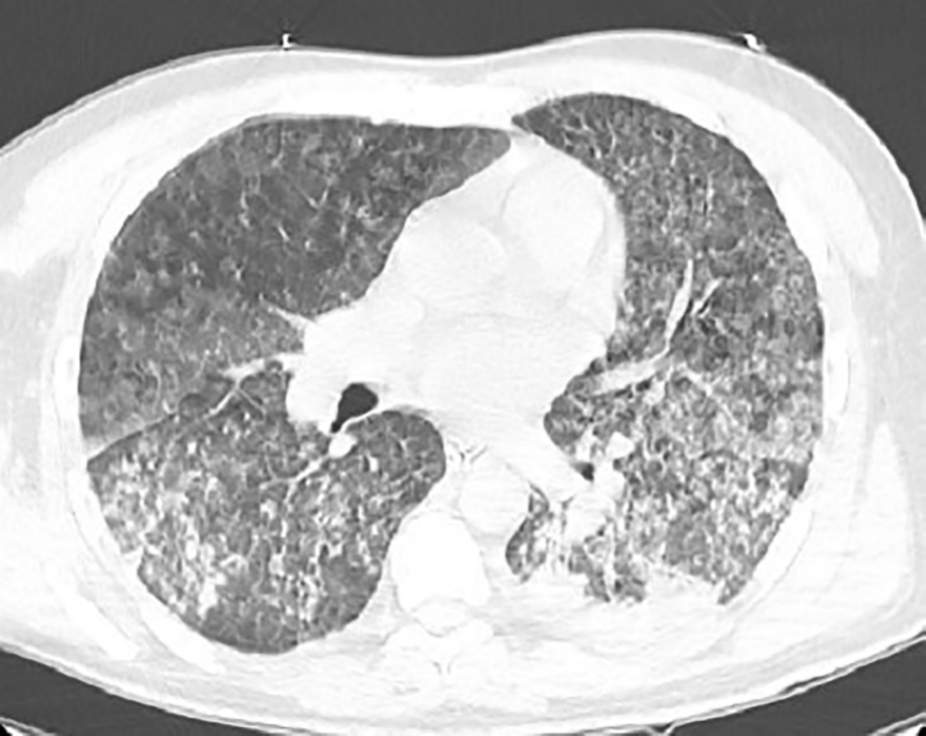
Chest CT Scan showing diffuse bilateral patchy alveolar infiltration with interlobular septal thickening and consolidation mostly seen at the posterior aspect of the lungs representing alveolar hemorrhage.
